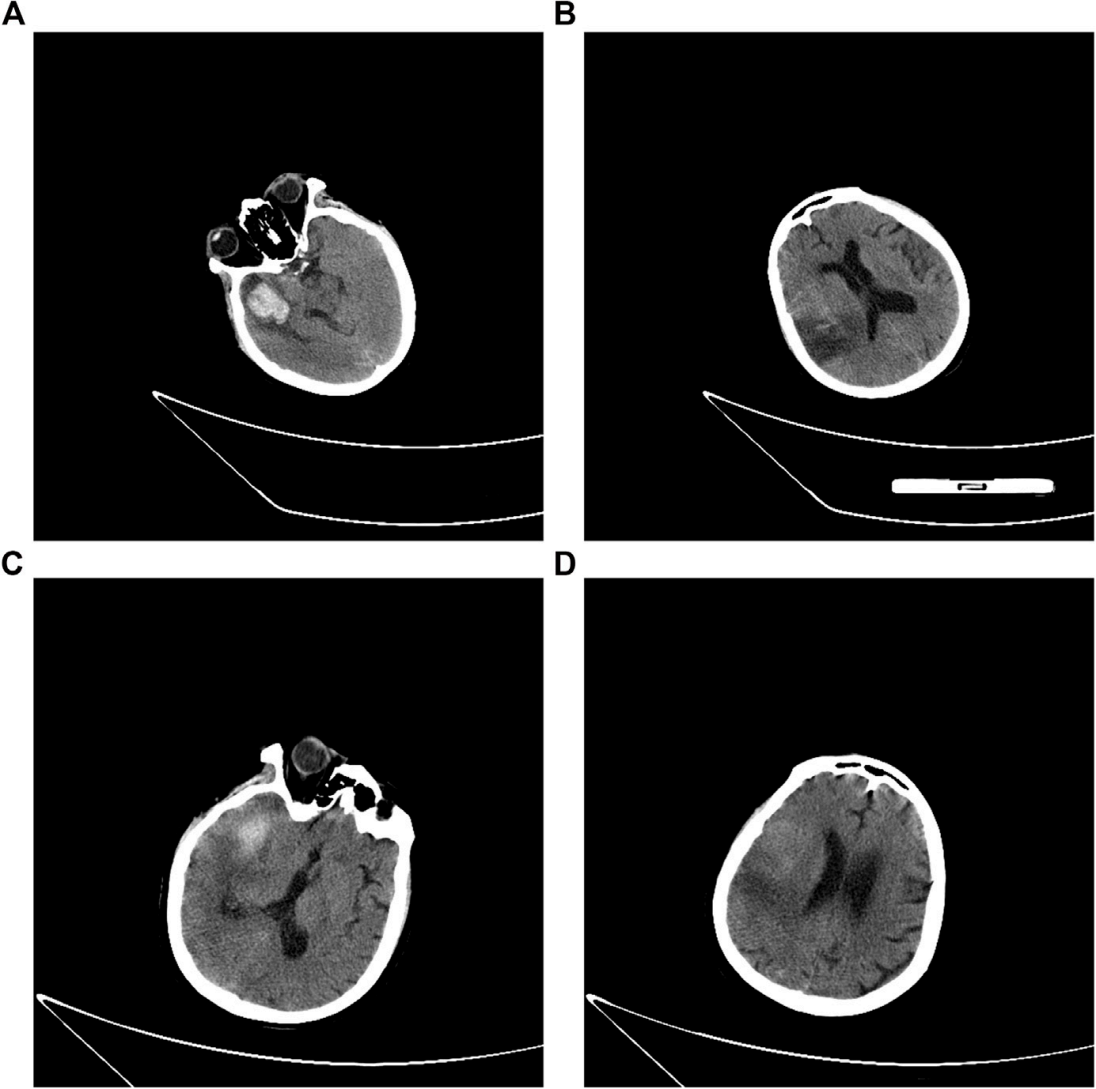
Head computed tomography scan on admission (A, B) and before discharge (C, D).
